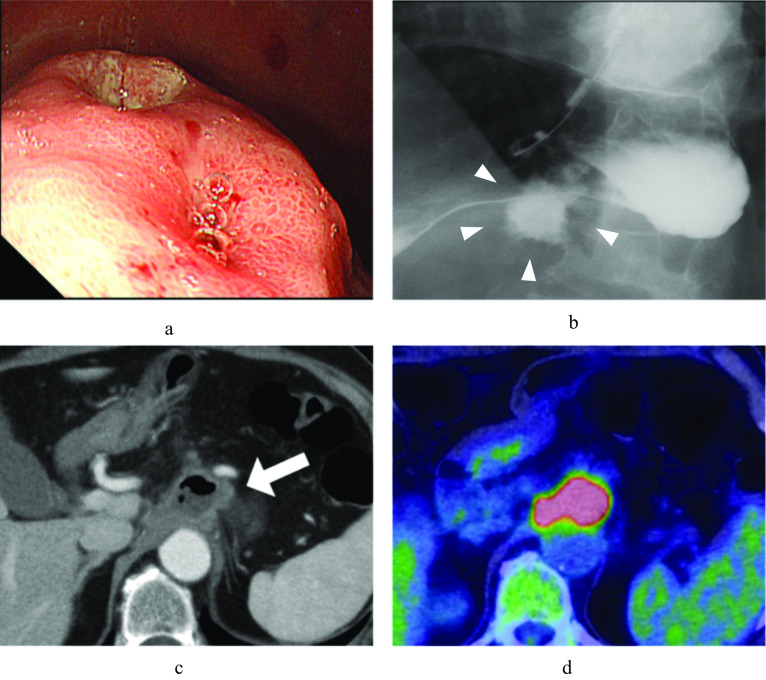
Submucosal tumor with depression was observed at the lesser-posterior side of the gastric wall (a). Leakage of contrast medium at the lesser side of the stomach (b). Cystic mass at the front of the celiac artery (c). FDG accumulation was observed at the cystic mass on positron emission tomography (d)
